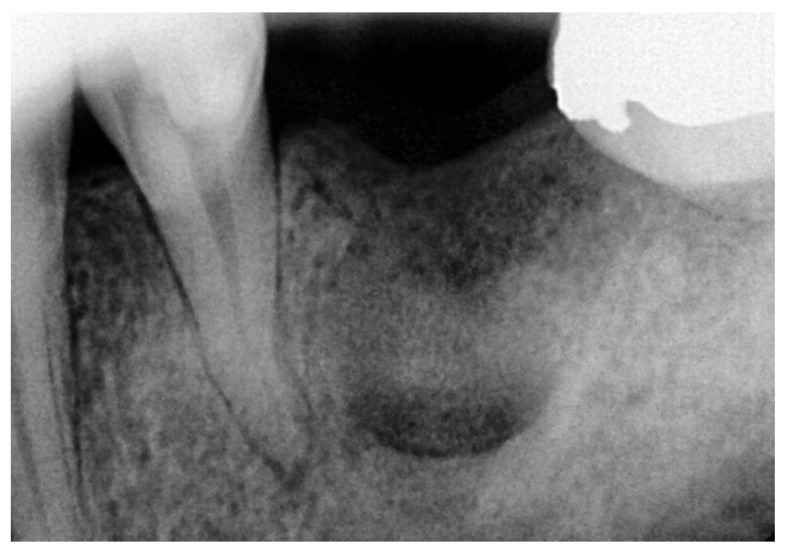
Postoperative periapical radiograph after 7 months (longest follow-up).
